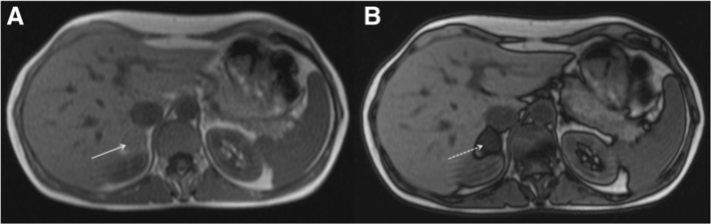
In-phase MRI image (a) showing a right adrenal lesion (solid white arrow). There is signal drop out (dashed white arrow) on the out-of-phase MRI sequence (b), confirming the presence of intracellular lipid and making the diagnosis of a benign lipid-rich adenoma
